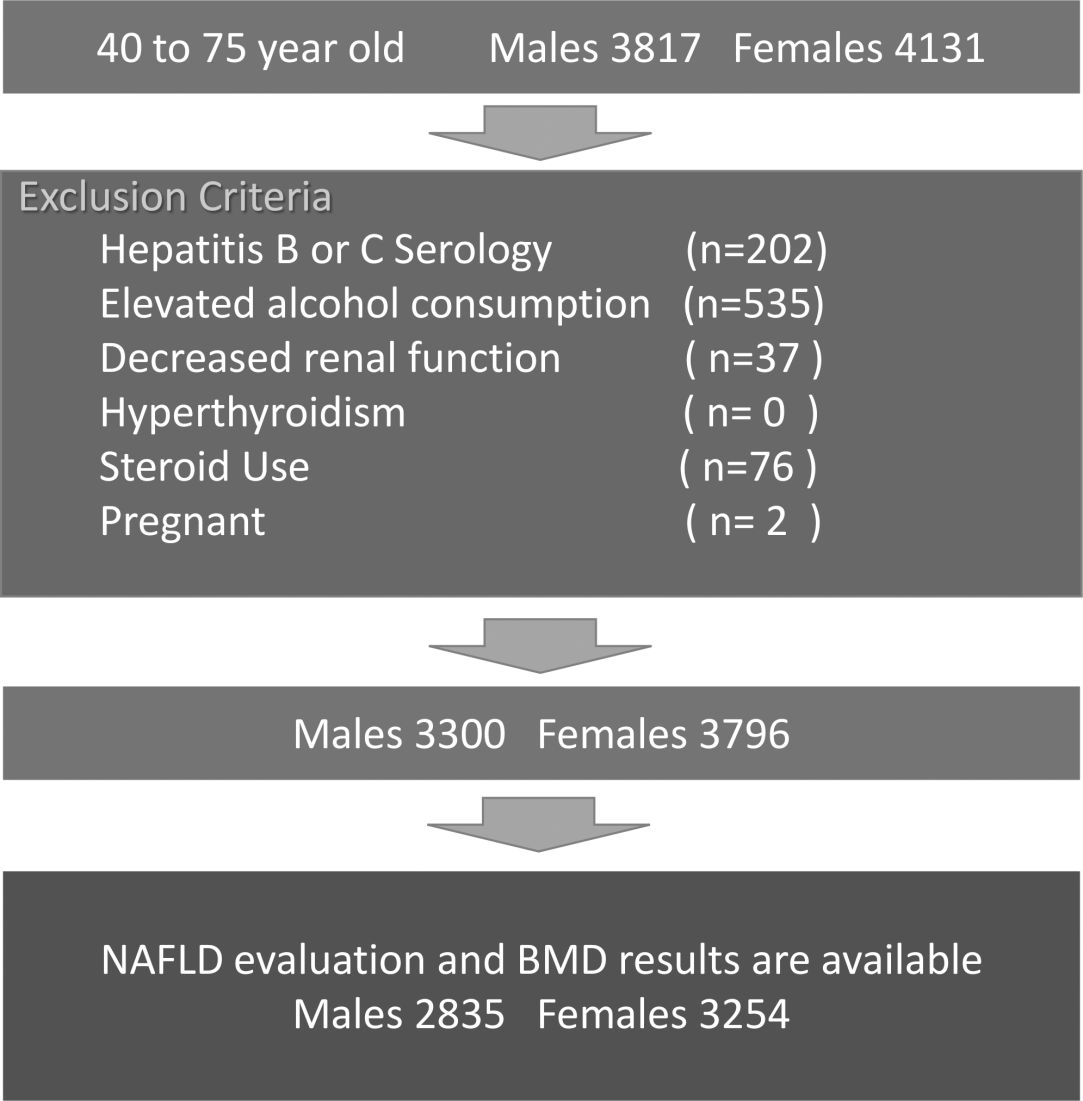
Participant selection. In the final step, 267 males and 266 females were excluded because of the lack of available ultrasonography results. 198 males and 276 females were excluded because bone mineral density results were not available.
